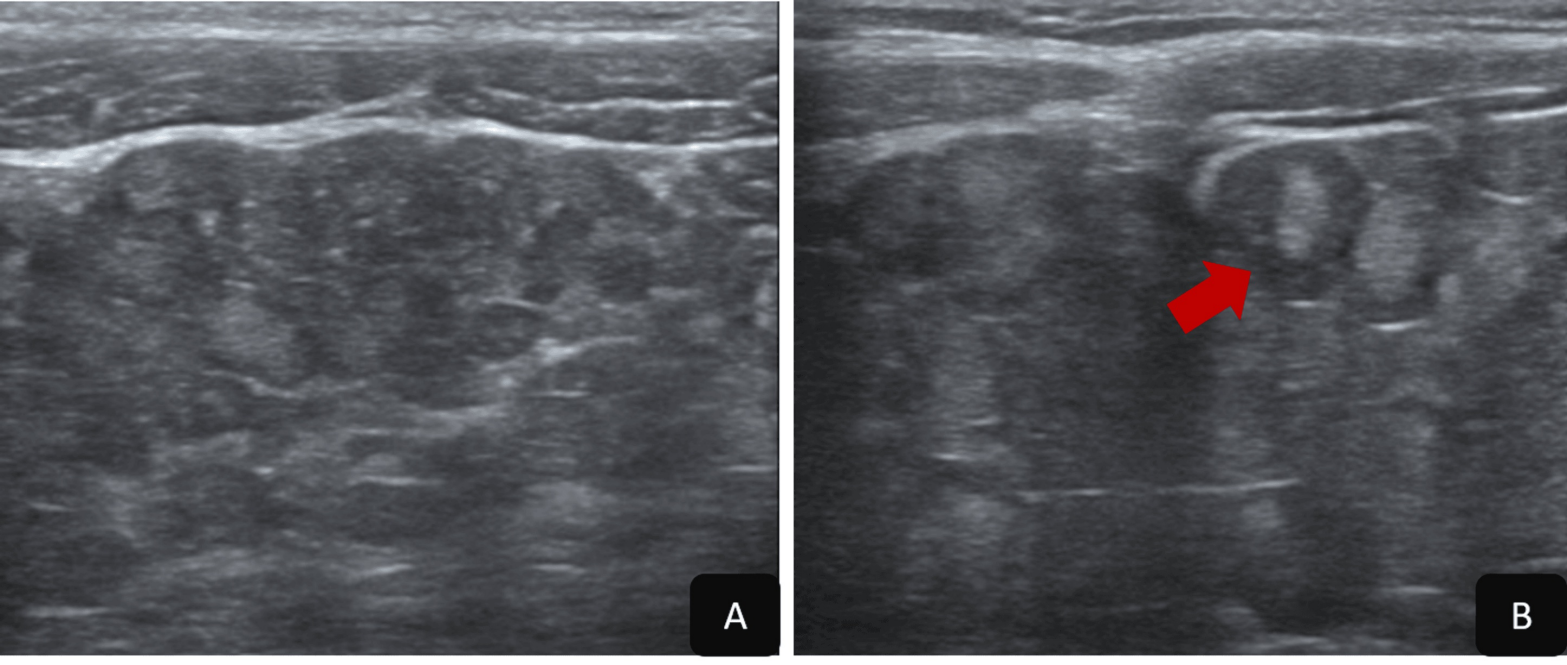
Abdominal ultrasound (A, B) Anterior mesenteric mass, relatively well-defined with lobulated contours, containing nodular structures with a hyperechoic center and a peripheral hypoechoic ring, producing a characteristic 'target' appearance (red arrow).
